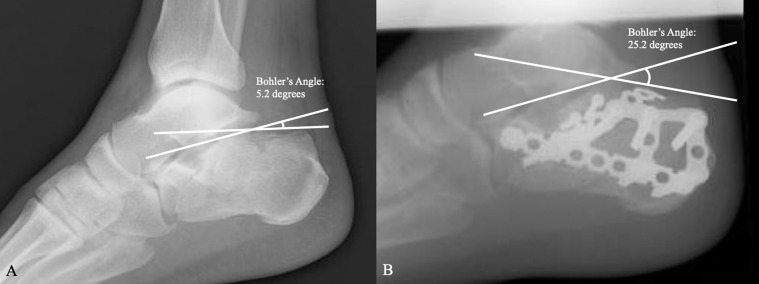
(A) Preoperative lateral foot radiograph of a comminuted, joint depression-type calcaneal fracture. Bohler’s angle measured 5.2 degrees through intersecting lines tangential to the superior tuber and the superior aspect of the posterior facet, and the superior aspect of the posterior facet and the anterior calcaneal process. In (B) a postoperative lateral foot radiograph is shown status post open reduction with internal fixation with a 3.5 mm lateral calcaneus plate with an additional two 2.7 mm lag screws underneath the posterior facet. There is restoration of Bohler’s angle measuring 25.2 degrees.
